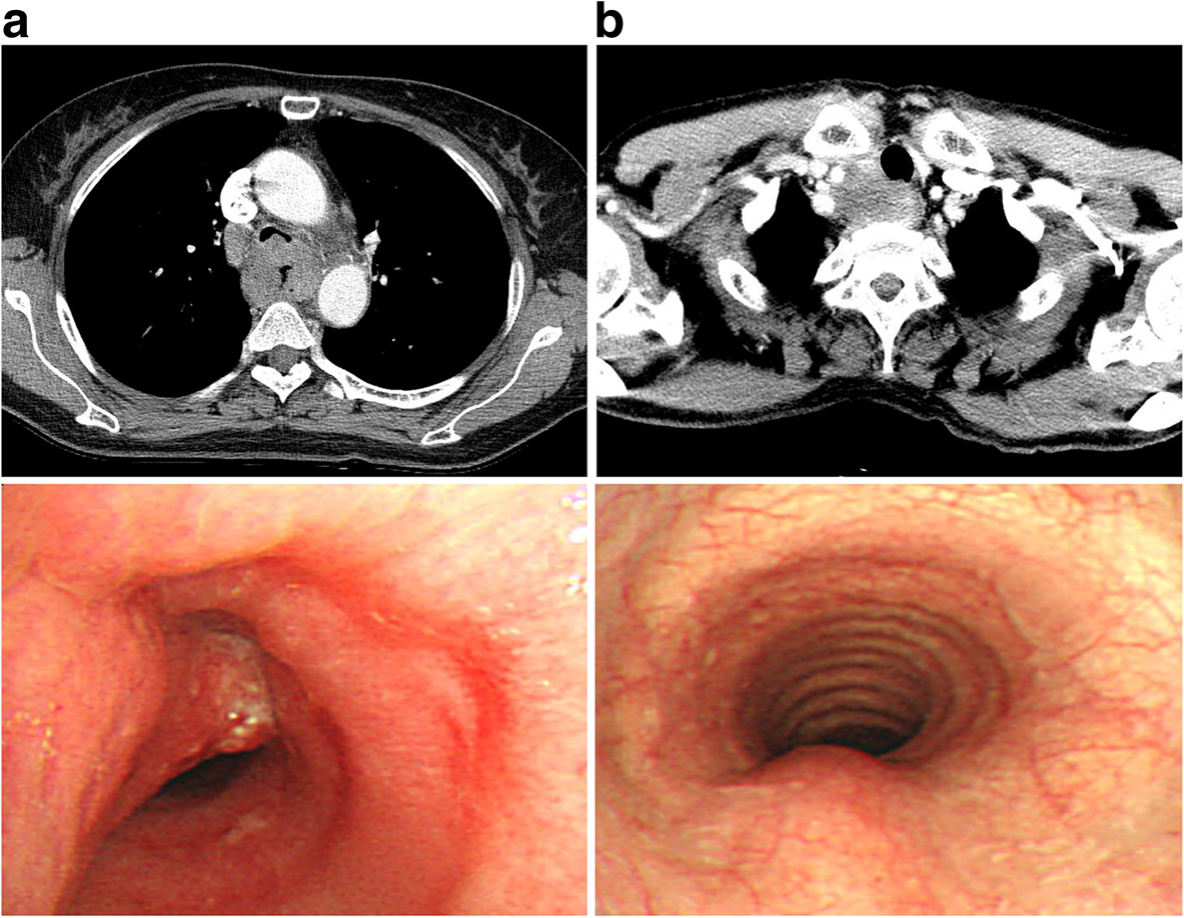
Chest CT and bronchoscopy images in patients with gross invasion (a) and mucosal change (b) on bronchoscopy
